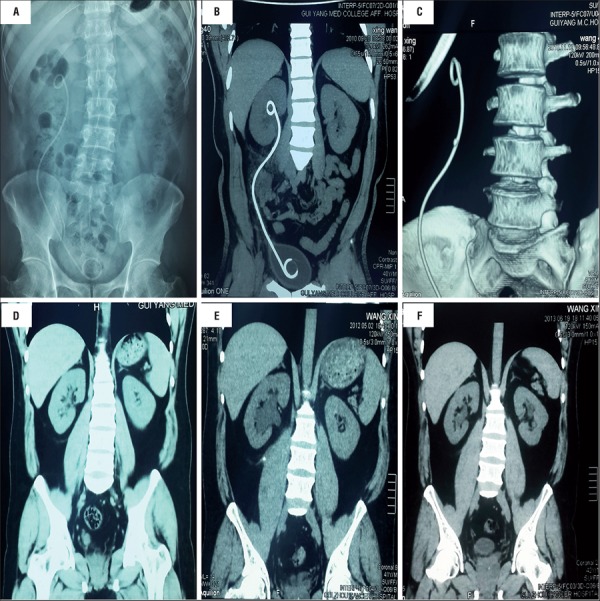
A and B) KUB and CT at one month; C) CT indicated that there was a stone like-material attached to the double-J tube at 3 months; D) CT indicated that there were no right hydronephrosis or other abnormalities at 5 months; E) CT revealed hydronephrosis secondary to a stone located in the upper ureter at 23 months; F) CT of urinary system revealed no hydronephrosis, renal atrophy or other complications at 34 months.
